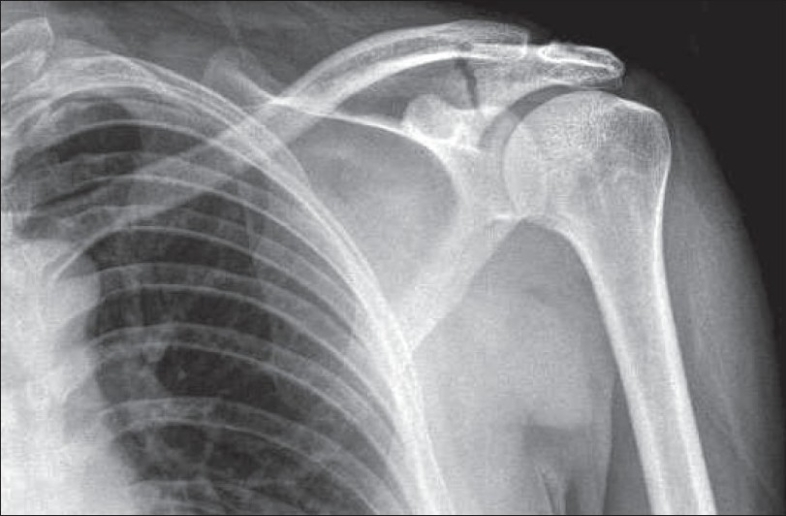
Initial radiograph of the scapular spine fracture
